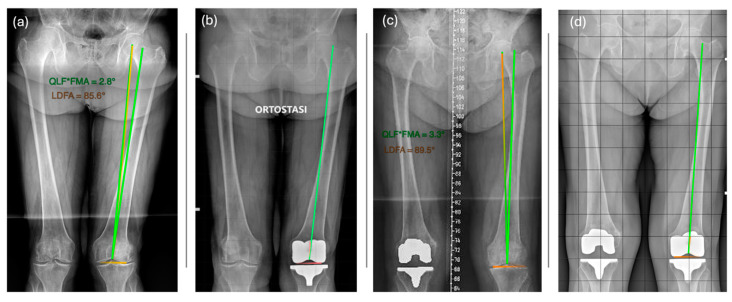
(a) In a patient with a QLF^FMA of 2.8° (green) and an LDFA of 85.6° (orange), we expected that the postoperative position of the trochlear groove of a femoral component with a PTA of 6° (orange) would be medial to the QLF (green) according to the mathematical formula of 1.2°. (b) Postoperative radiograph of the same patient, in which the angle measured on the long-leg radiograph of the lower limb was 1.2° medial to the QLF. (c) In a patient with a QLF^FMA of 3.3° and an LDFA of 88.5° we expected that the postoperative position of the trochlear groove of the femoral component with a PTA of 6° (orange) would be lateral to the QLF (green) according to the mathematical formula of 1.2°. (d) Postoperative radiograph of the same patient, in which the angle measured on the long-leg radiograph of the lower limb was 1.2° lateral to the QLF.
